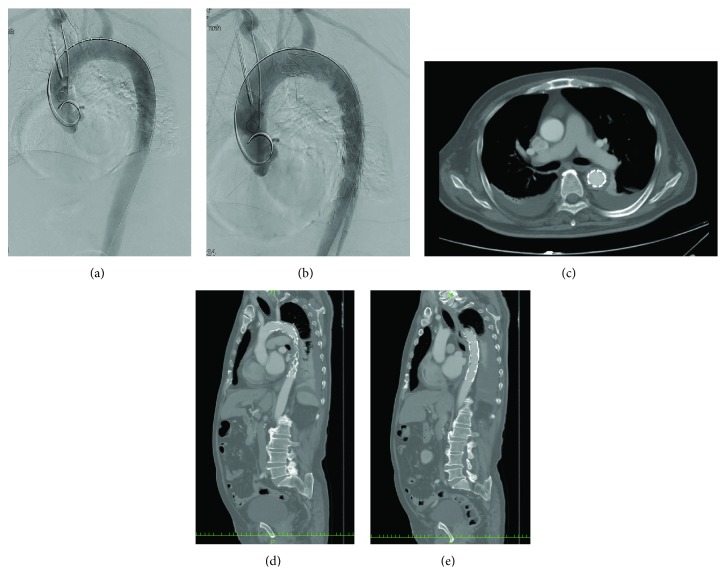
Case 2. Intraoperative aortography: (a) before deploying the stent graft and (b) after deploying the stent graft. There were no endoleaks. Postoperative computed tomography performed one month after the procedure: (c) axial image, (d) sagittal image, and (e) sagittal image in another slice. The thickness of the intraluminal hematoma had decreased.
